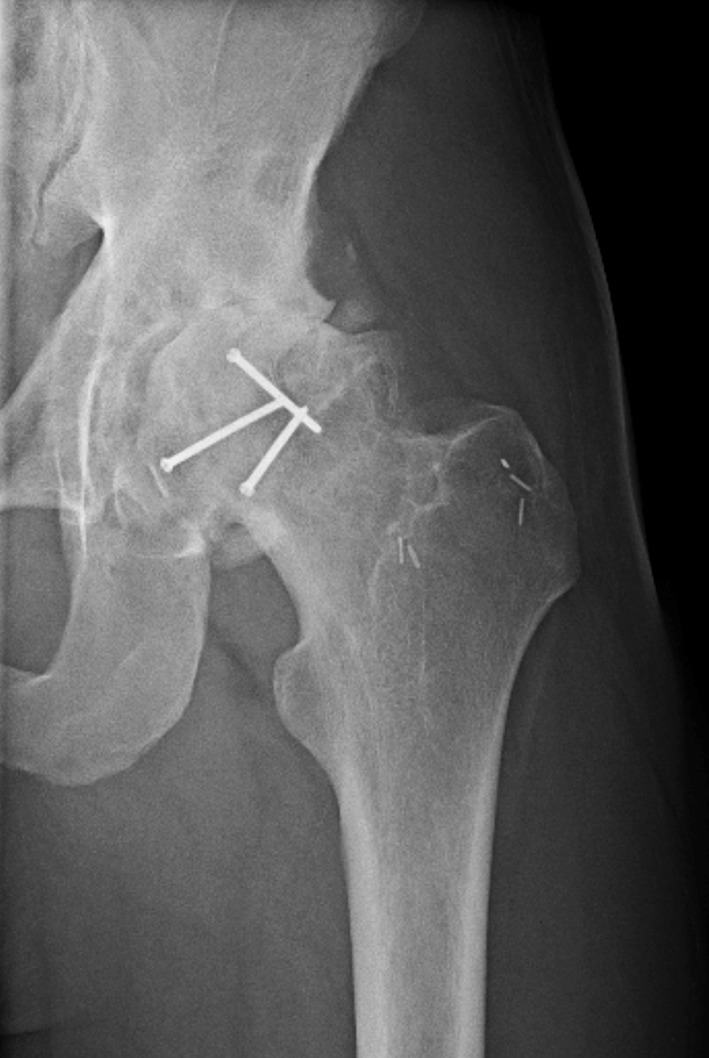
Follow-up radiograph of a 19-year-old male 4 years after left femoral head operative reduction and internal fixation (ORIF) with end stage post-traumatic arthritis in the hip joint
